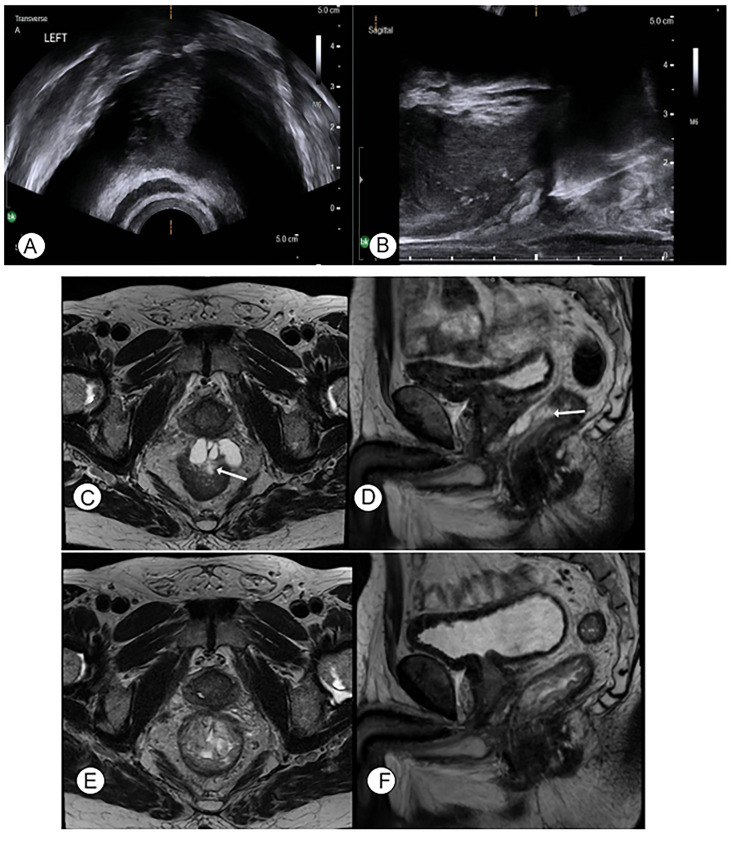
(A, B) Ultrasound imaging at the time of the procedure. (C, D). Treatment planning MRI scan showing rectal wall infiltration (white arrow). (E, F) Repeat treatment planning MRI scan showing resolution of rectal wall infiltration after hyaluronidase injection. *Abbreviation:* MRI = magnetic resonance imaging.
